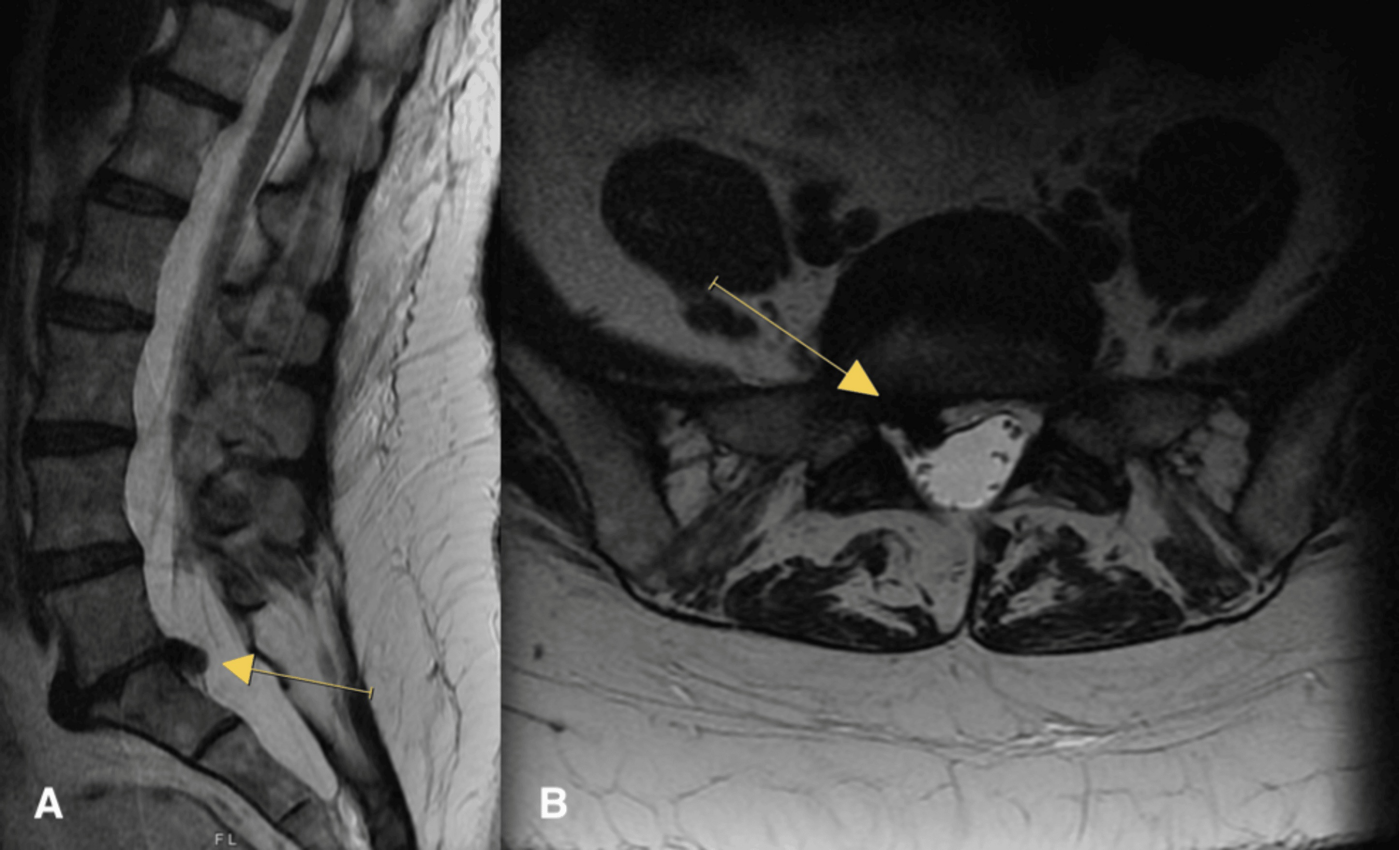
MRI of the lumbar spine demonstrating disc herniation at L5-S1 (yellow arrows). (A) sagittal view; (B) axial view
